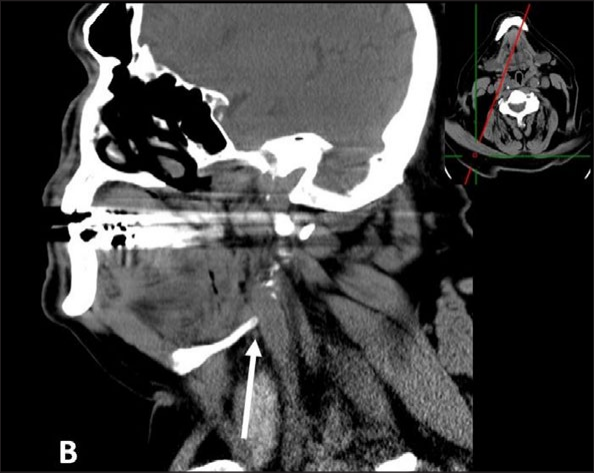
Reformatted non-enhanced oblique sagittal CT-scan image of the neck (soft tissue window) showing the greater horn of the hyoid bone (arrow) abutting the anterior wall of the distal right common carotid artery.
